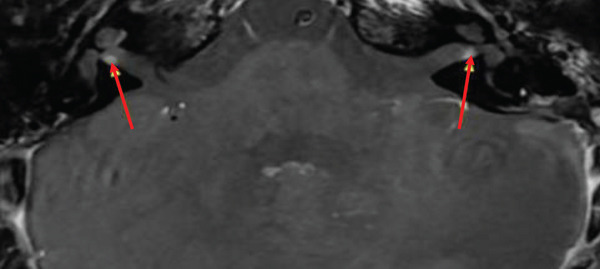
Contrast‐enhanced T1‐weighted MRI shows symmetric foci of enhancement in the fundi of bilateral IACs (two red arrows). Physiologic enhancement of Scarpa′s ganglia is favored when the findings are symmetric and centered at the fundus, versus the asymmetrical location, size, and morphology typically seen in vestibular schwannomas.
